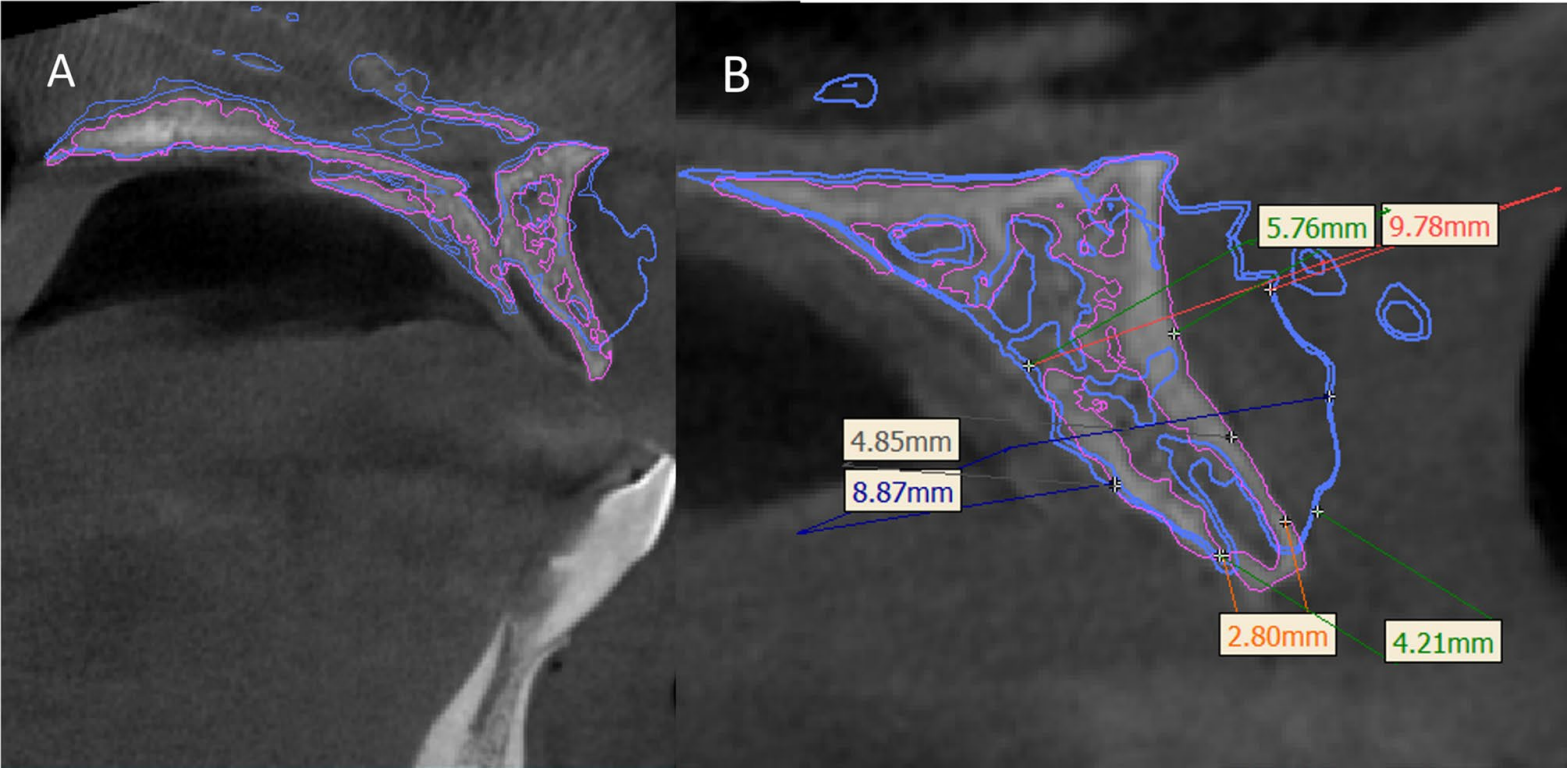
A) Standardization of assessment method for calculation of bone width by superimposition of preoperative CBCT (pink outline) and the 4 months post-operative CBCT (blue outline) B) bone width was measured at three different levels; at the crestal, at 5 mm and 10 mm
